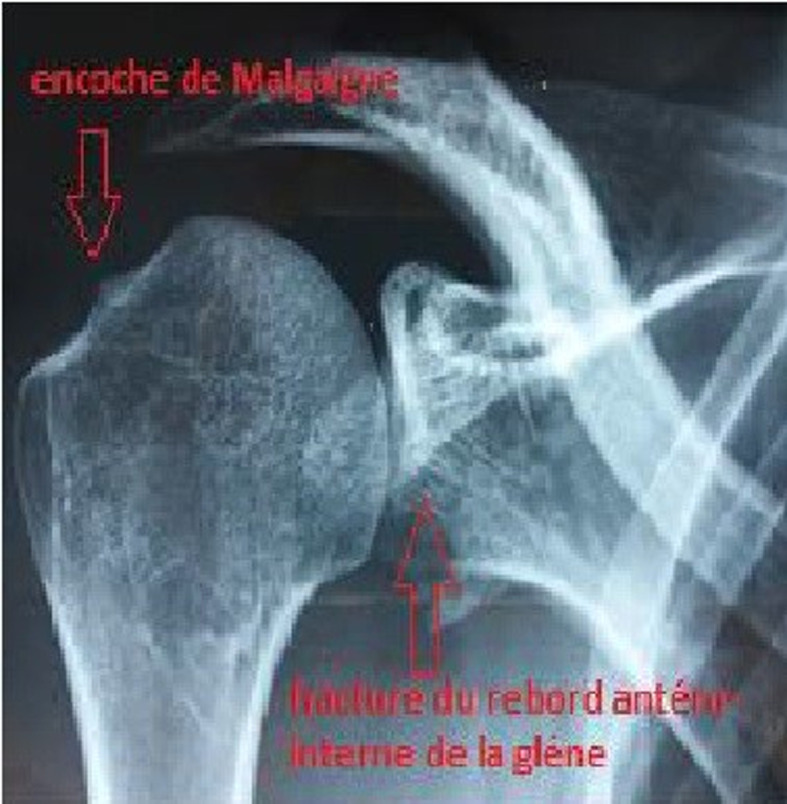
radiographie épaule face en rotation neutre montrant une fracture de rebord antérieur de la glène avec encoche de Malgaigne
